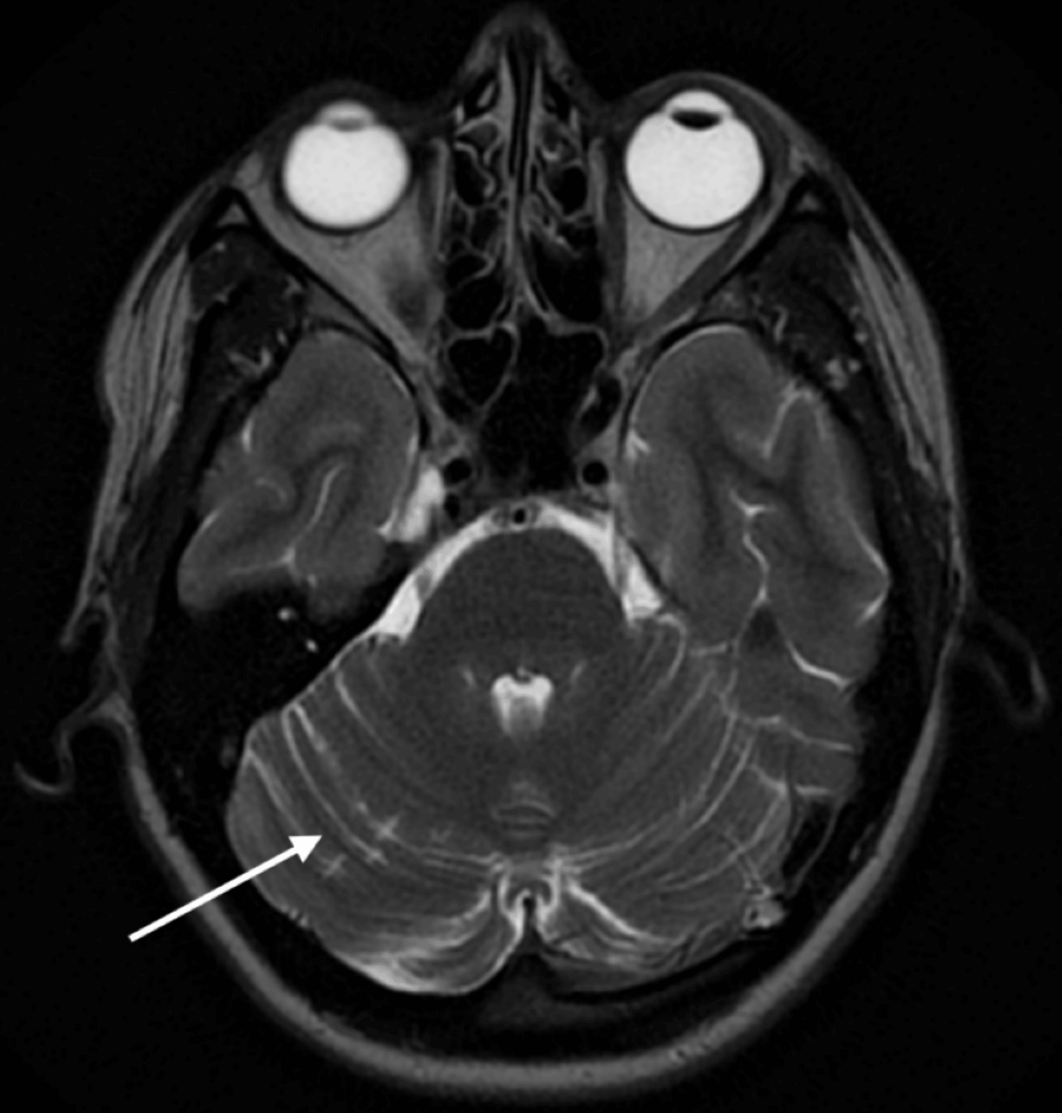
MRI Head (Axial view) demonstrating right sided cerebellar infarcts.
